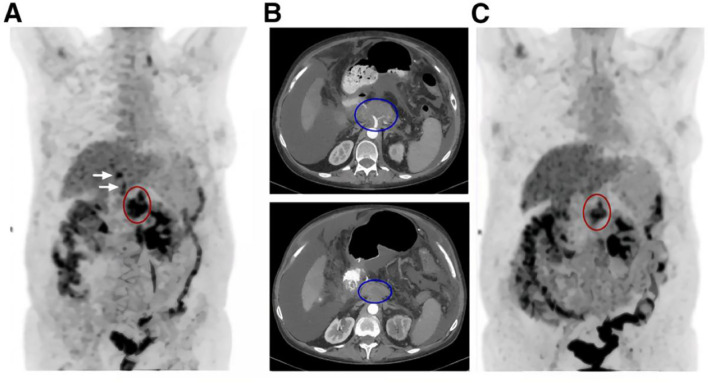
^18^F-FDG PET and CT scans of patient 3 before [(A, B) upper panel] and after [(B) lower panel, (C)] [^177^Lu]Lu-3BP-227 therapy. (A)
